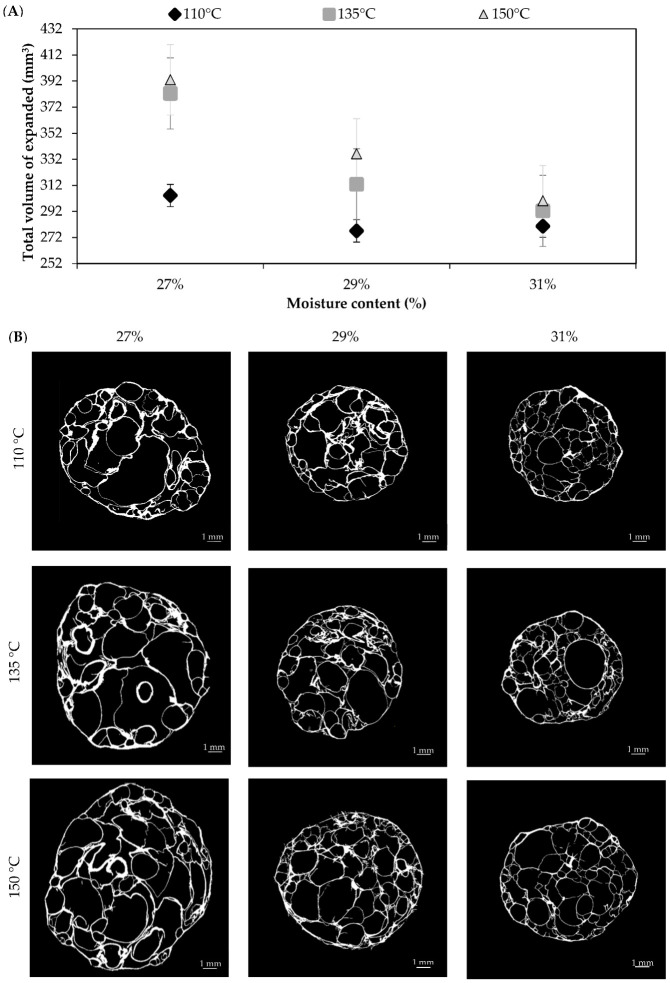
(A) Total volume of the expanded products obtained after microwaving (800 W) pellets for 50 s, which were produced feeding a rice-flour mix with different moisture levels (27%, 29%, or 31%) and processed using different extrusion temperatures (110 °C, 135 °C, or 150 °C). Results are expressed as mean (*n* = 3) ± typical error. (B) Cross-section X-ray image of the matrix volume of expanded products (MVE). The scale bar represents 1 mm.
